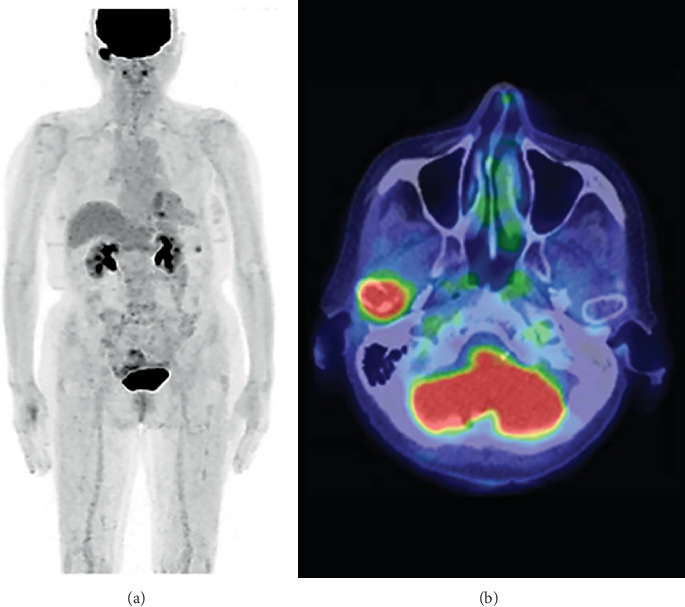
Results of ^18^F-fluorodeoxyglucose positron emission tomography and computed tomography (FDG-PET/CT). (a) Whole-body FDG-PET/CT. (b) Axial FDG-PET/CT. Maximum standardized uptake value was 7.62 in the right mandibular condyle, and abnormal FDG uptake was observed in the left eight rib and fourth thoracic vertebra.
